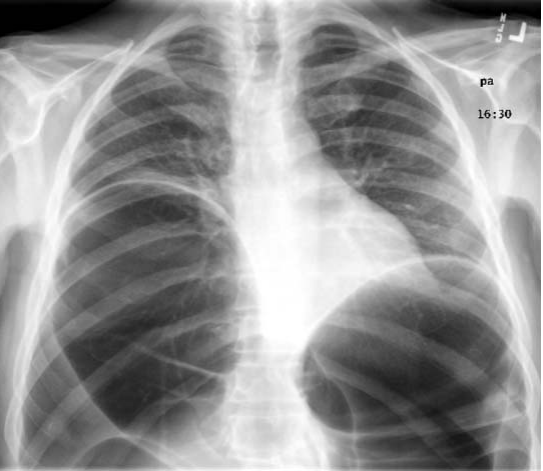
Admission chest radiograph demonstrating elevation of both hemidiaphragms.
